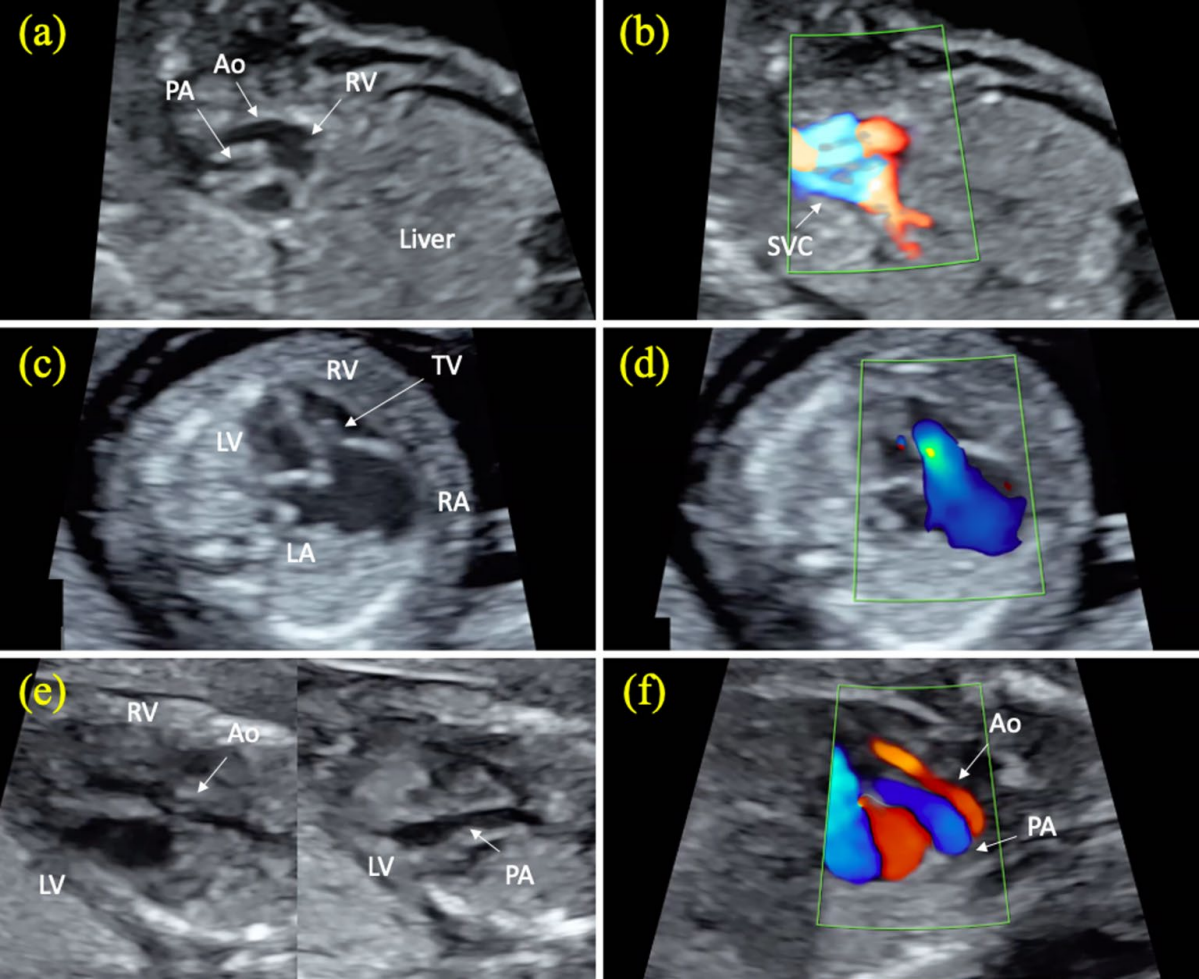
First trimester fetal echocardiographic images of double outlet right ventricle, Ebstein anomaly, and transposition of the great arteries. Double outlet right ventricle (a, b). Gray scale (a) and color Doppler (b) view of the aorta and pulmonary artery originating from the right ventricle. Note that the aorta and pulmonary artery are not crossed. Ebstein anomaly (c, d). In gray scale, the tricuspid valve is located more apically in the four‐chamber view, and the left atrium is dilated (c). Severe tricuspid regurgitation with apical onset on color Doppler in the same fetus (d). Transposition of the great arteries (e, f). In gray scale, the aorta originates from the right ventricle, and the pulmonary artery originates from the left ventricle (e). Color Doppler image of the parallel course of the aorta and pulmonary artery in the sagittal oblique section in the same fetus (f). The aortic valve is dysplastic, and there is reverse flow in the aorta. Ao, aorta; LA, left atrium; LV, left ventricle; PA, pulmonary artery; RA, right atrium; RV, right ventricle; SVC, superior vena cava; TV, tricuspid valve.
